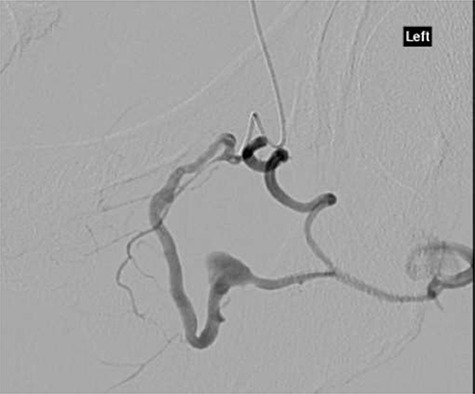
CT angiography with dye showing dilatation of collateral branch of left obturator artery.
